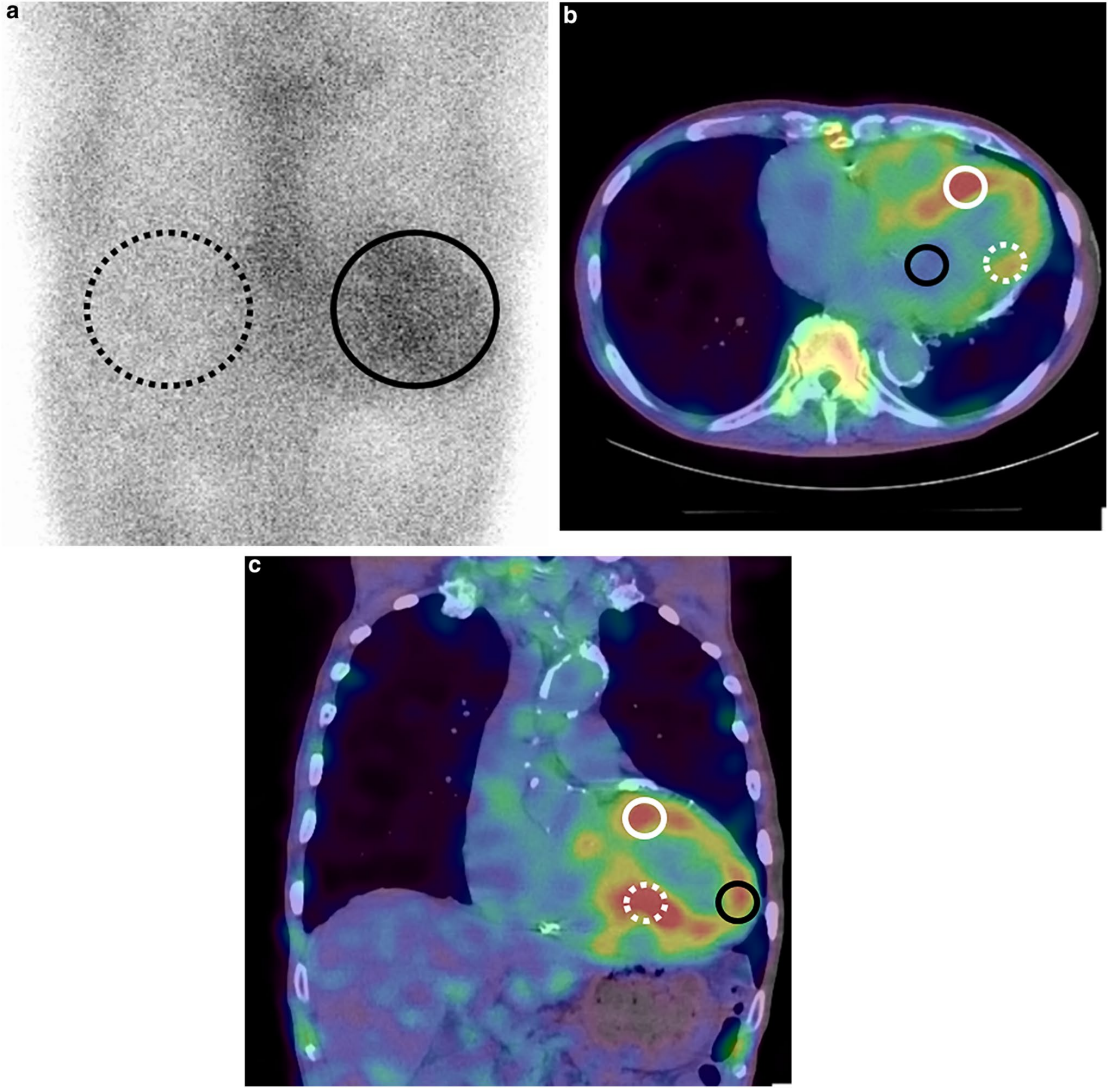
ROIs setting in PYP planar and SPECT/CT images. On planar image (A), a circular ROI was put on the heart (black circle) and mirroring it to the contralateral chest (black dashed circle) to calculate H/CL. On SPECT/CT fusion images (B, C), a circular ROI was set at the maximum count area of PYP accumulation in five regions of the left ventricular myocardium and at the minimum count area in the left ventricular cavity (B; black circle) on axial image. The ROIs were set for the septal (B; white circle) and lateral regions (B; white dashed circle) on the axial image and apical (C; black circle), anterior (C; white circle), and inferior walls (C; white dashed circles) on the coronal image (C)
